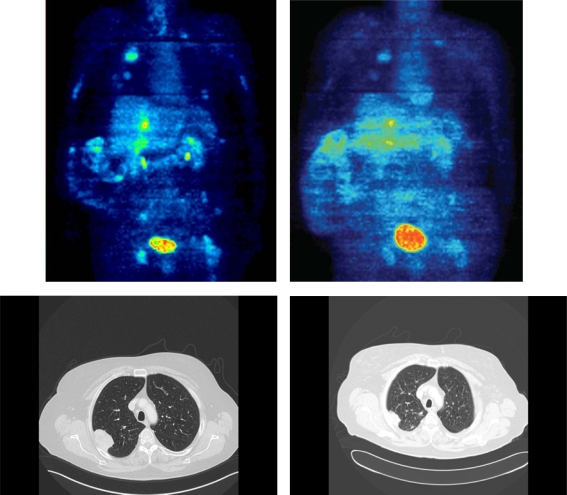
Case of a 62 year old female with a leiomyosarcoma, grade III, of the vena cava with lung and liver metastases. FDG PET showed a decrease of the average SUV from 6.7 and of the SUV_max_ from 13.5 (*upper left image*) to 3.5 and 6.1 (*upper right image*), respectively, as well as a decline of the FDG uptake in all evaluable lesions (mSUV PET images, colour-scales are directly comparable (0–10 SUV)). As an example, the corresponding CT scan showed the reduction in size of one reference lesion in the lung demonstrating a PR according to RECIST criteria (*lower left and right images*). Additionally, liver metastases demonstrated a partial remission (not shown).
